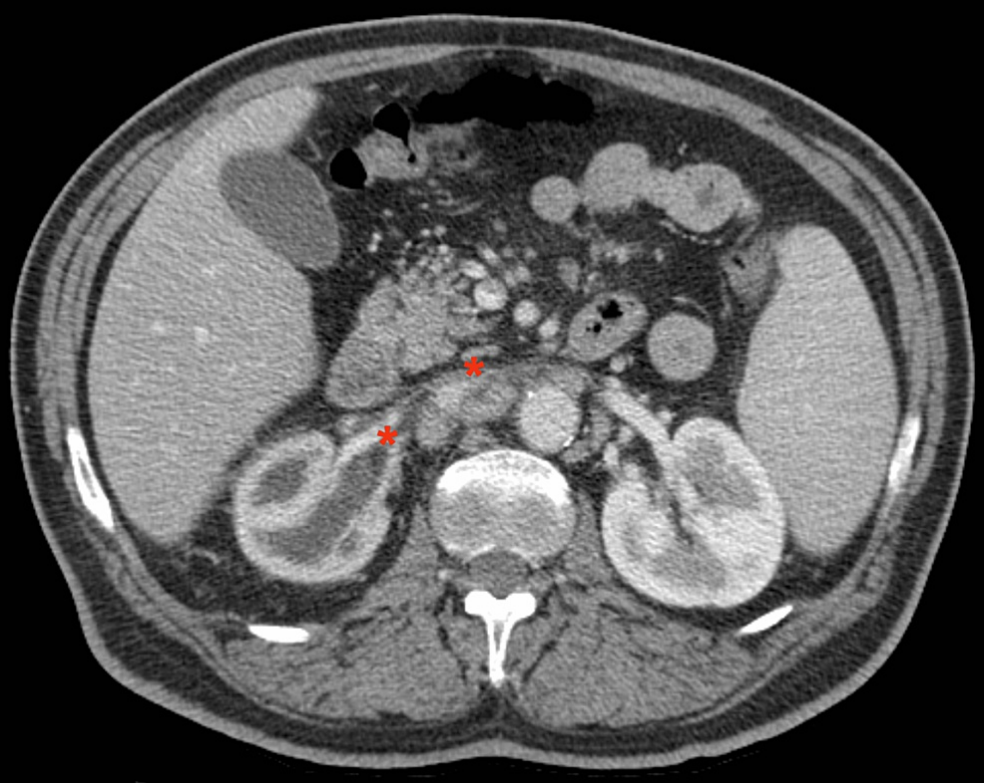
Abdominal CT-scan revealing right renal atrophy with nonspecific ureteral and pyelocaliceal ectasia and retroperitoneal fibrosis involving the right ureter
